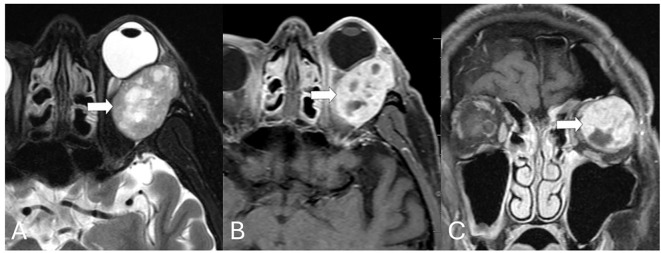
A 55-year-old man with a left lacrimal adenoid cystic carcinoma. (A) Axial T2 MRI shows a cystic and solid appearance of the left lacrimal mass (arrow). (B, C) Axial and coronal T1 post-contrast MRI shows an oval-shaped mass with enhancement of the solid component (arrows).
